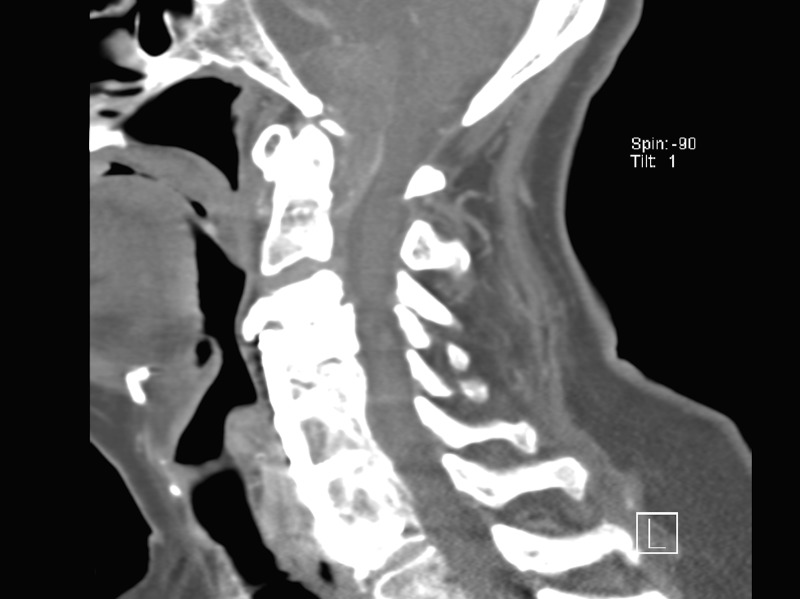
February Sagittal CT Image Cervicomedullary junction
